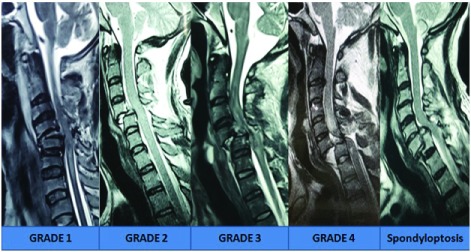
MRI images showing various types of traumatic subluxation, as per the Meyerding grading
